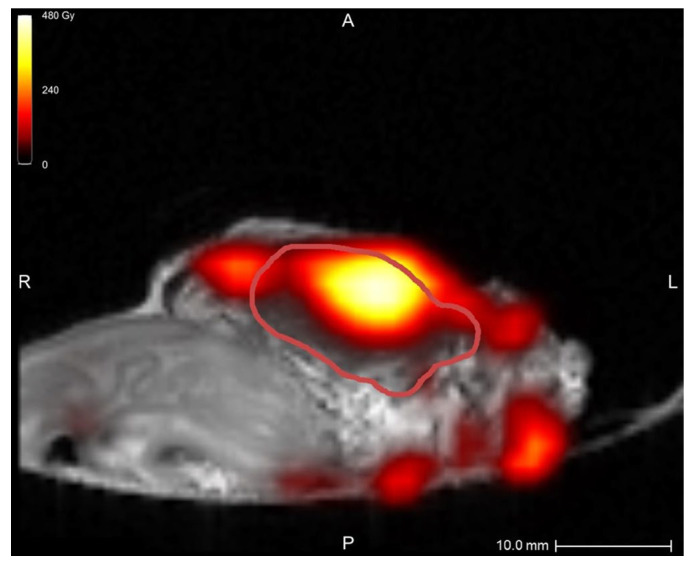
MRI quantification after nonradioactive intratumoral ^165^HoMS injection in sample 5, with a theoretical specific activity of 12.00 MBq/mg ^165^HoMS. The red line delineates the tumor. The highest dose, at the location of the ^165^HoMS deposition, was observed within the tumor. Multiple MRI artifacts caused inaccurate dose estimations surrounding the tissue, which were created by air and heterogeneous tissues. This figure was created in Q-Suite (version 2.1).
